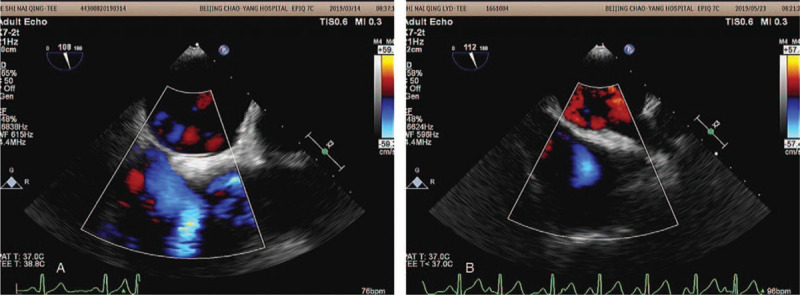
Interatrial septum on TEE of a patient with recurrent AF. TEE result at the first admission before ABS. TEE result at 6-month follow-up with no shunt between 2 atrias. TEE = trans-esophageal echocardiography, AF = atrial fibrillation, ABS = atrial balloon septoplasty.
